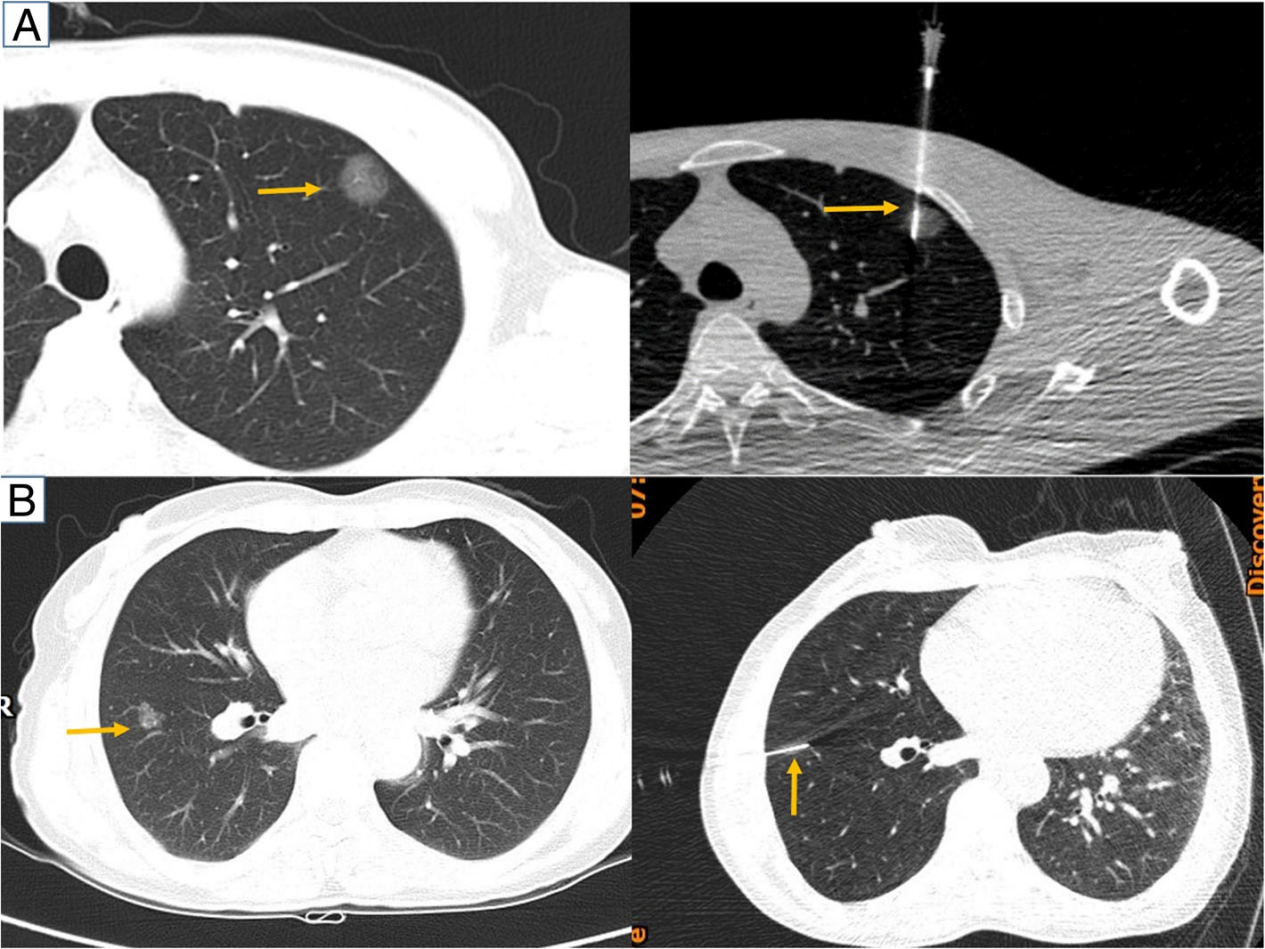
Computed tomography-guided semi-rigid single hook wire localization. a, b An initial CT was performed to determine the puncture site. After the localizing needle was inserted into the lung, a CT scan was obtained for confirmation (Yellow arrows). b The wire was located 2.5mm higher than the nodule
